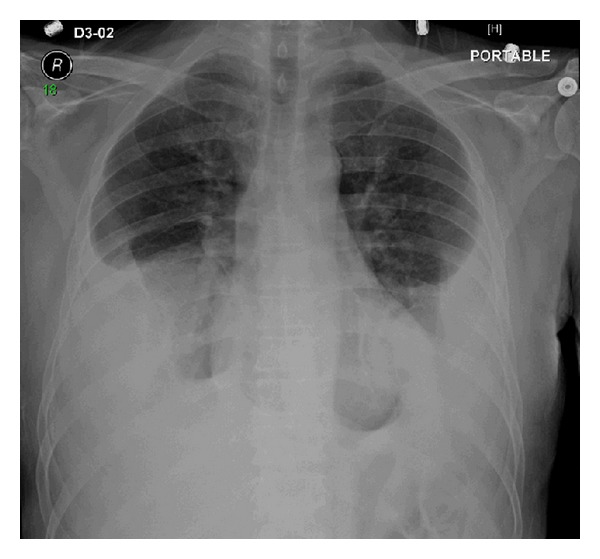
A chest plain radiograph (X-ray) showing bilateral pleural effusion, partial bibasilar atelectasis, and linear pleural calcifications involving the right lung.
